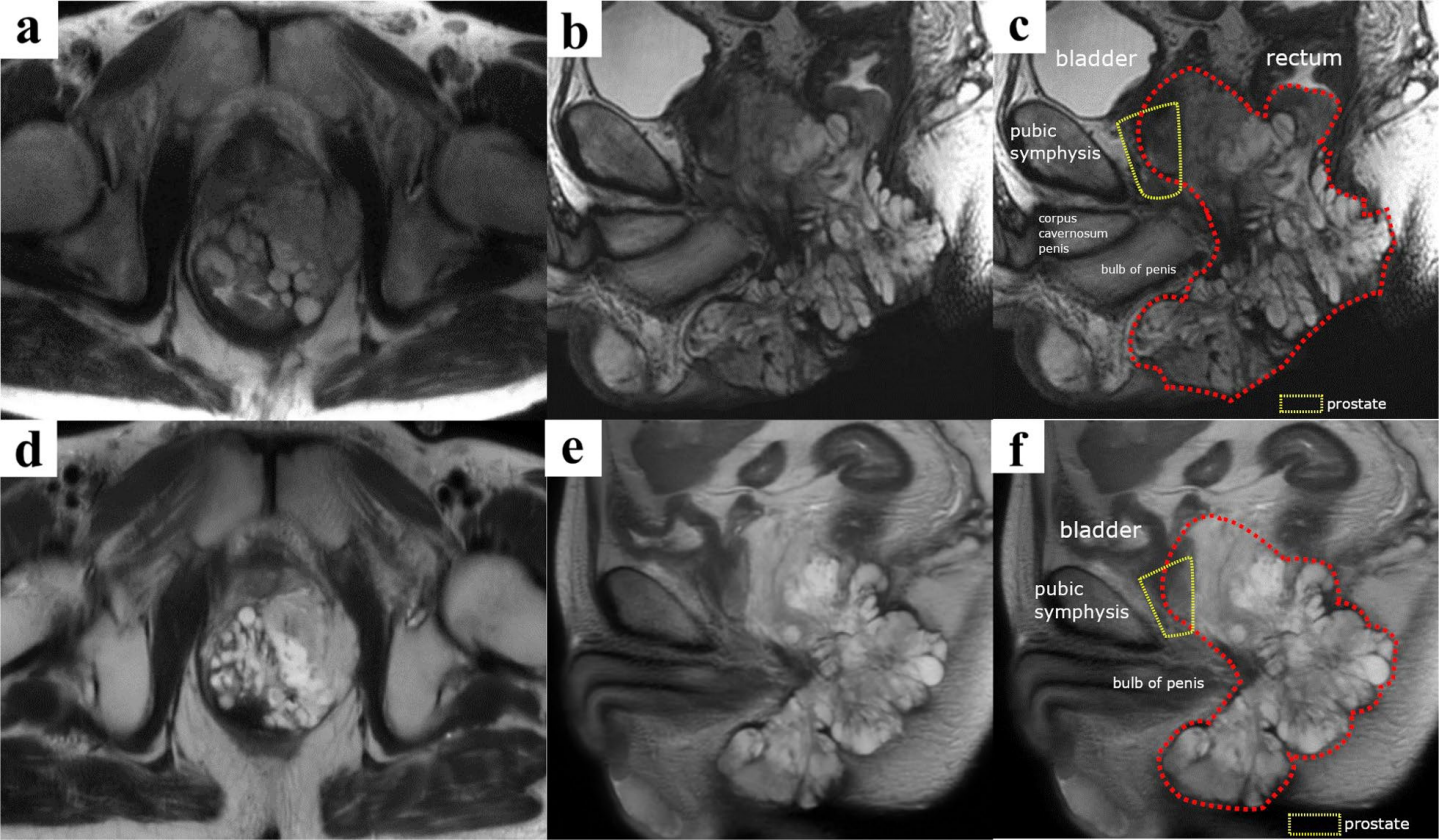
T2-weighted magnetic resonance imaging of the anorectal fistula cancer. Infiltration into the prostate, seminal vesicles, levator ani muscle, and left internal obturator muscle was revealed in the region surrounded by the red dotted line (a–c). After chemoradiotherapy, the tumor had slightly shrunk with stable disease as defined by the revised RECIST guidelines (version 1.1) (d–f)
